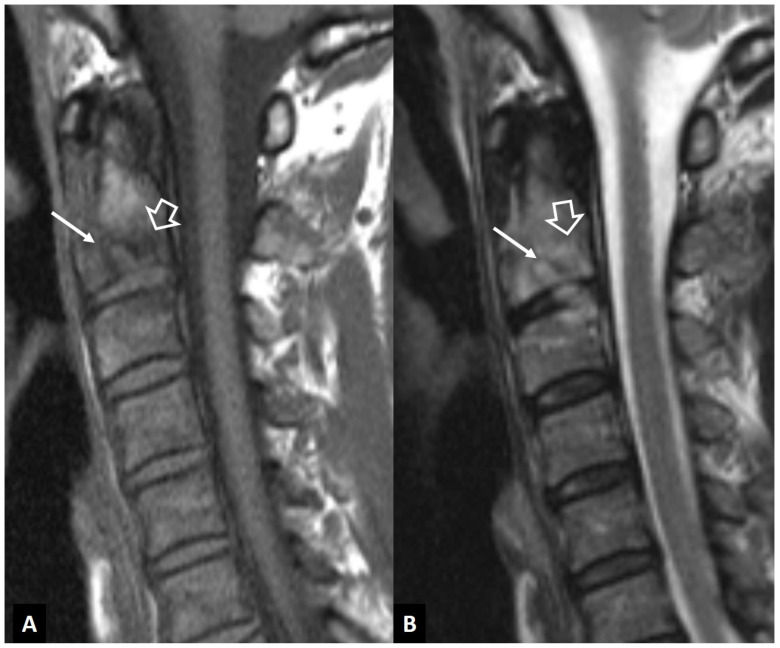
A 48-year-old male elite biker with intense pain during training. Sagittal T1-w (A) and STIR (B) MR images showing the Schmorl’s node within the C2 vertebra (thin arrows) and the surrounding bone marrow edema (open arrows). Clinical improvement was achieved with rest, painkillers, and NSAIDs over 3 weeks.
